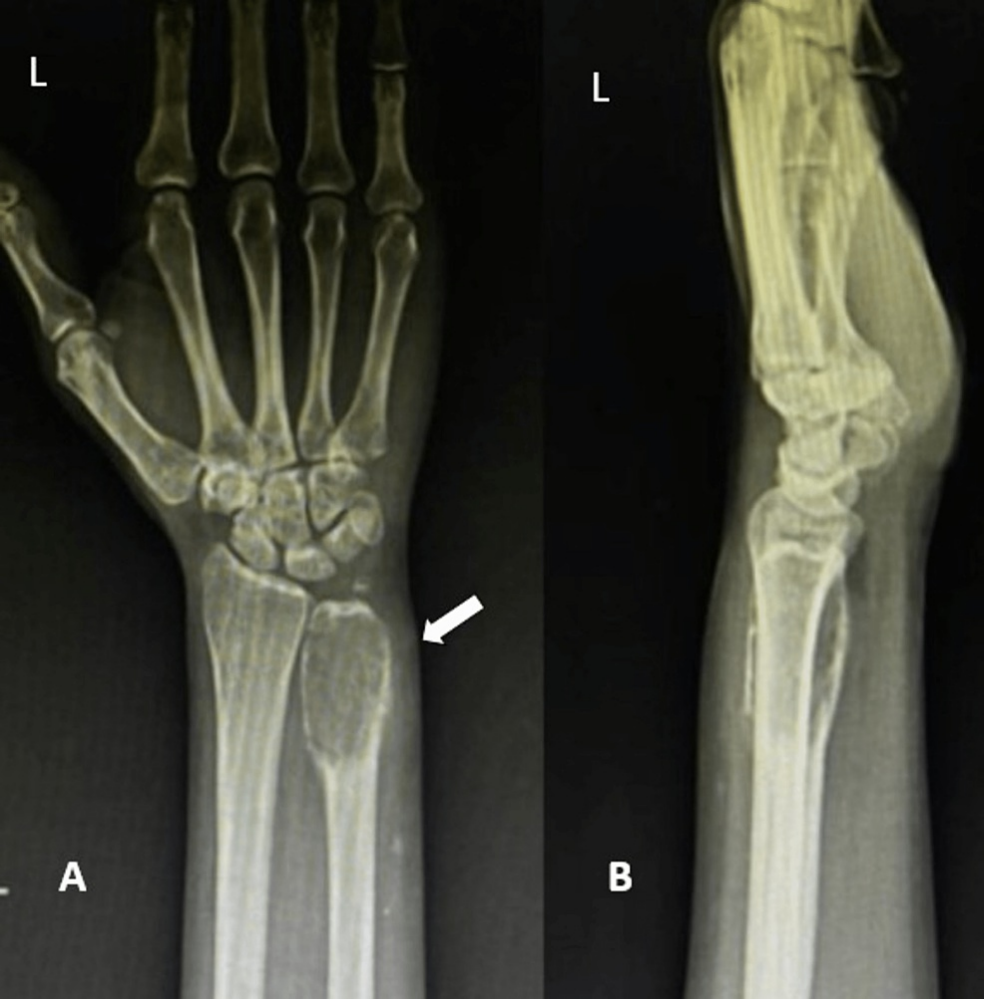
X-rays of the left wrist in AP and lateral views A and B show X-rays of the left wrist in anteroposterior and lateral views showing a radio-lucent and multi-lobar area with clear margins in the distal ulna (marked with an arrow). AP: Anteroposterior
